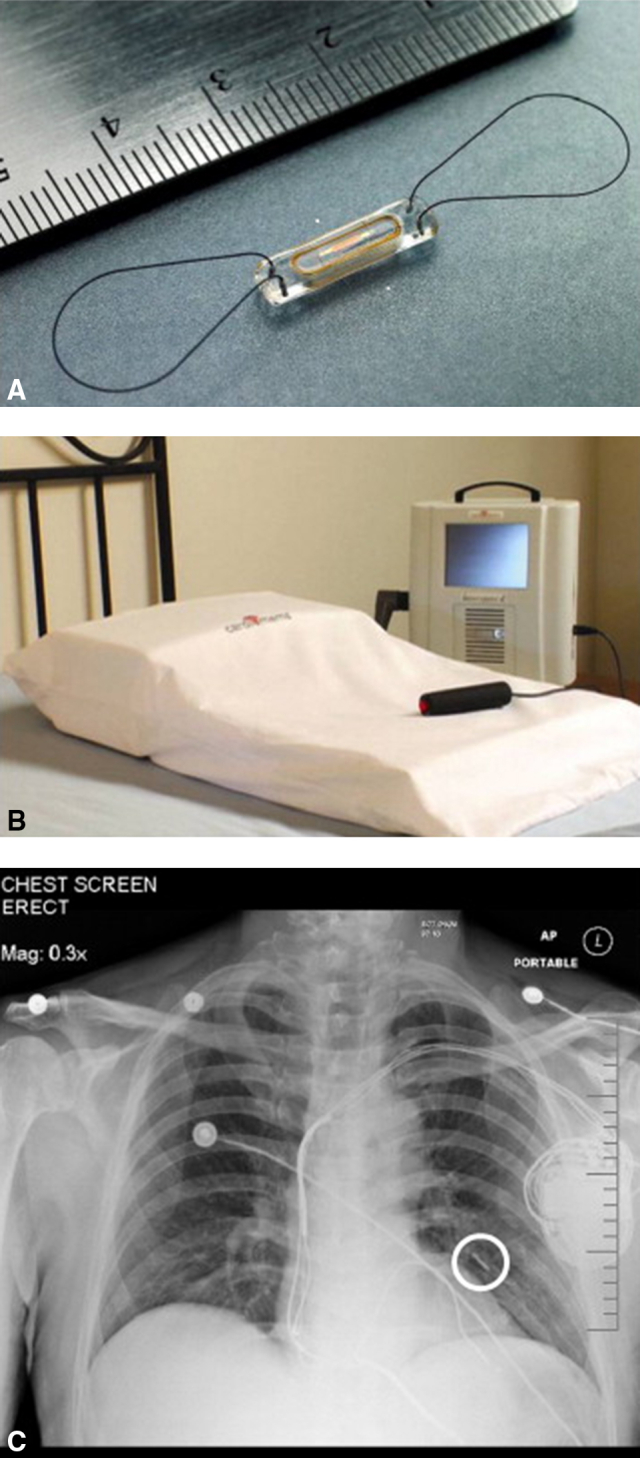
CardioMEMS (Abbott, Abbott Park, Ill) noninvasive hemodynamic monitoring system. A, Close-up view of the CardioMEMS device. B, Wireless pressure monitoring system. C, Chest radiograph of the position of the CardioMEMS device in the left pulmonary artery (*circle*).
